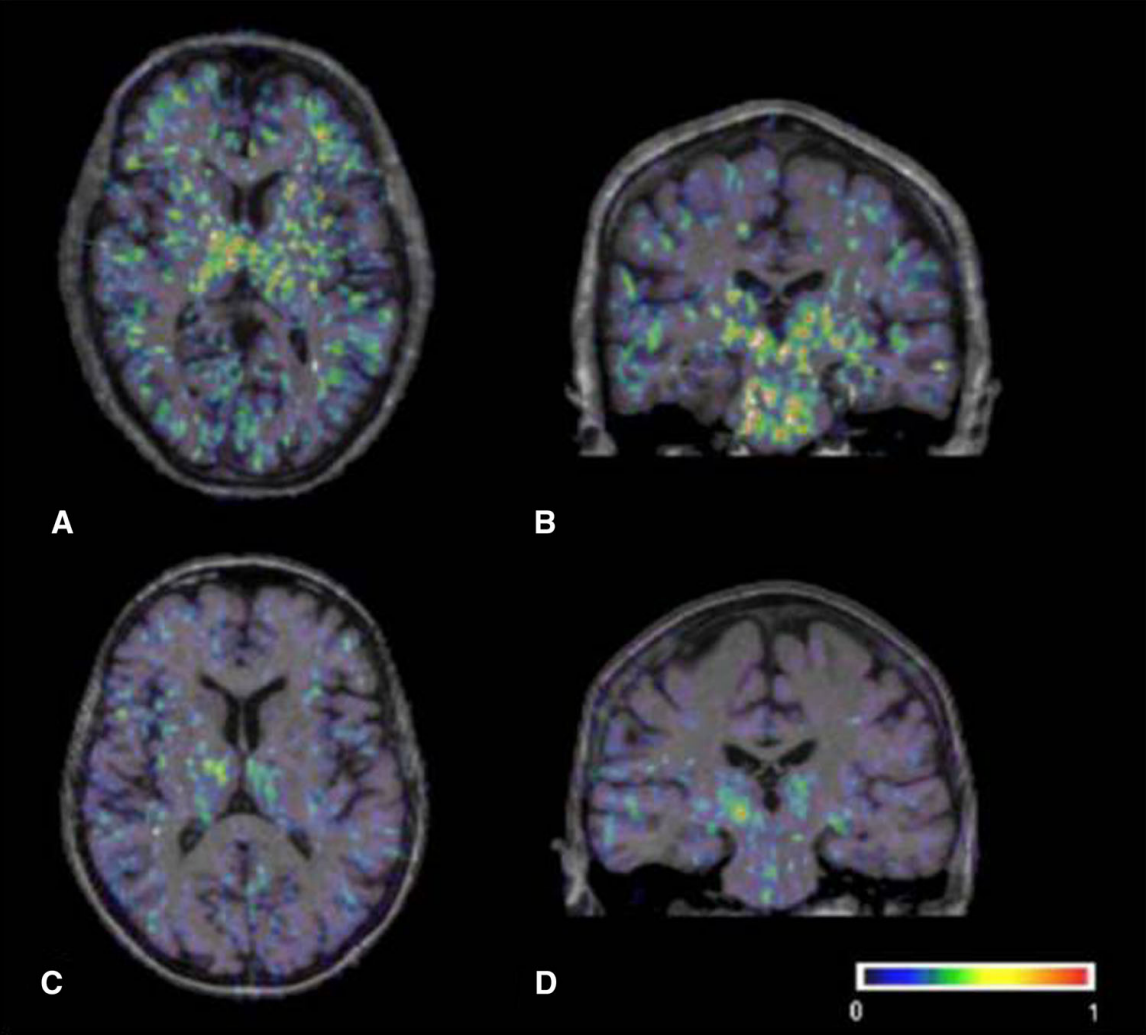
Transverse and coronal sections of binding potential maps coregistered to the individual MRI. In the PD patient (a, b), binding is increased in the basal ganglia, pons and frontal regions, while the healthy control person (c, d) only shows constitutive [^11^C](R)-PK11195. Binding in the thalamus and pons. The color bar denotes binding potential values from 0 to 1. Modified with permission from [31]
